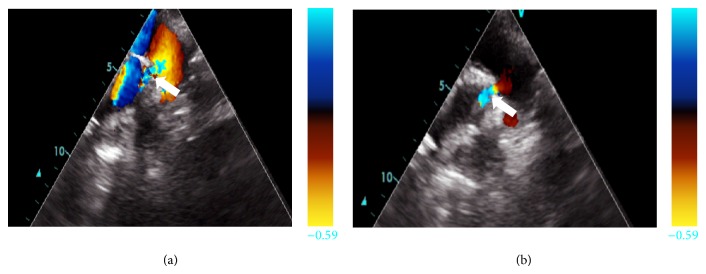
Colour doppler images from the transthoracic echocardiogram show right-to-left shunt through the subaortic VSD (arrow).
